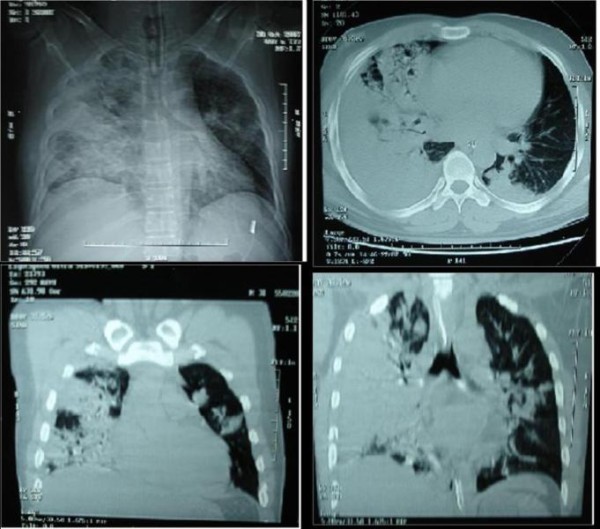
CT thorax showing features suggestive of necrotizing pneumonia.
